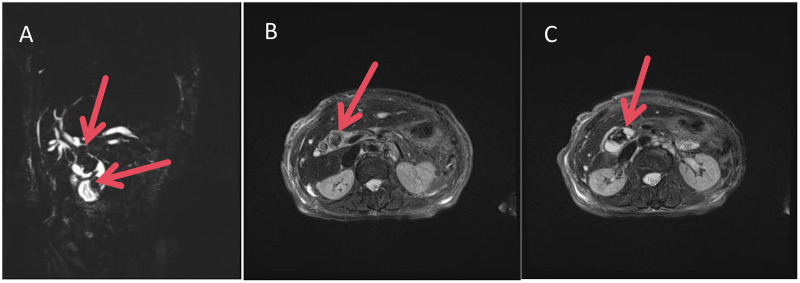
Preoperative upper abdominal MRI. (A) MRCP image demonstrating diffuse dilatation of the intra- and extrahepatic biliary tree with multiple filling defects. (B) Axial T2-weighted image confirming nodular filling defects in the common bile duct. (C) Another axial T2-weighted image (different slice) showing additional filling defects with red arrows indicating areas of abnormality in the biliary tree.
